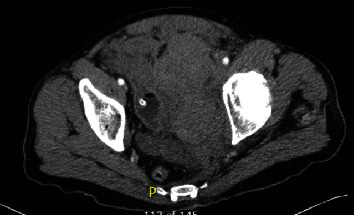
Initial CT abdomen and pelvis in ED (axial): 16 hours postop with large pelvic hematoma.
